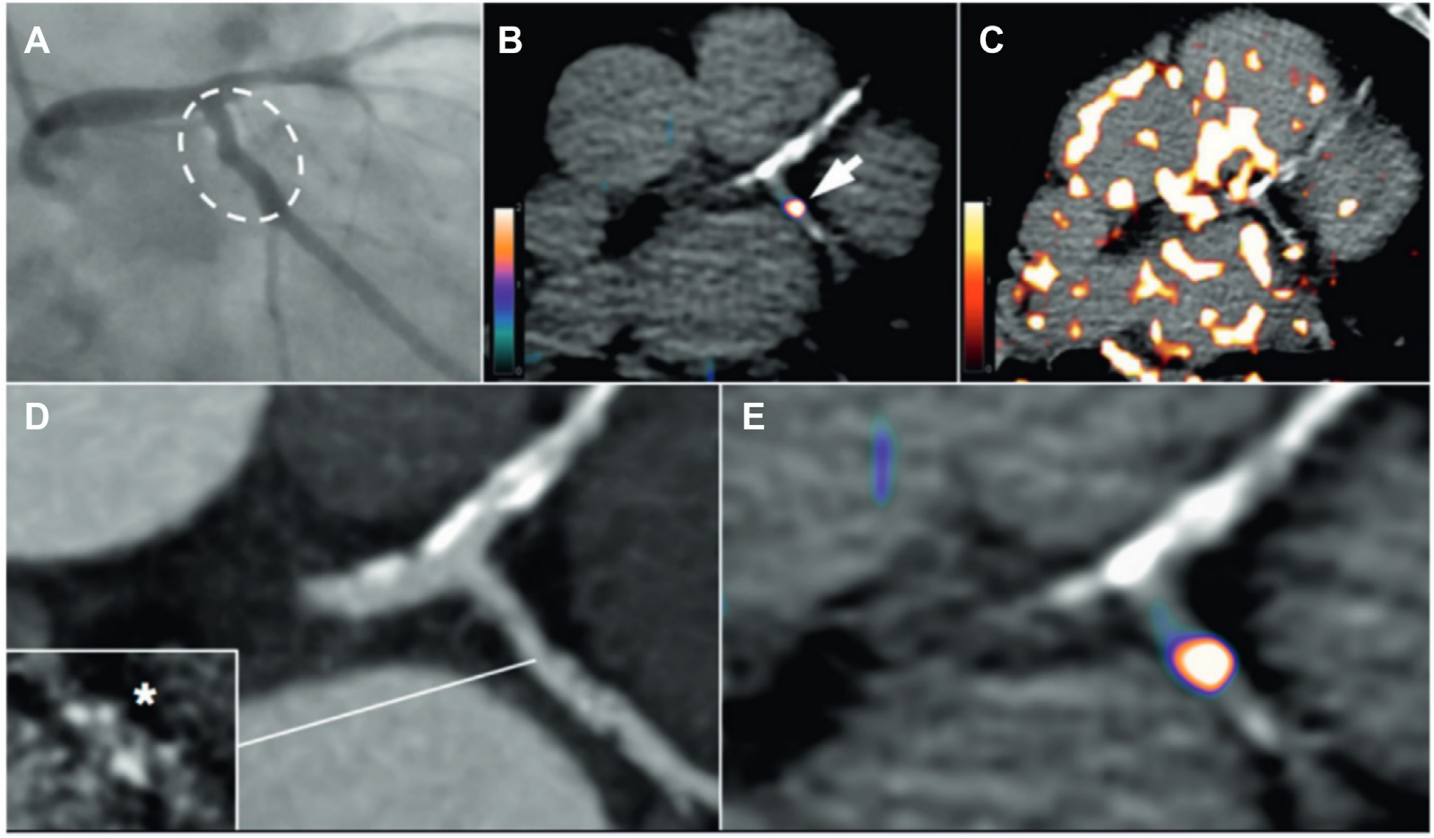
Coronary Artery PET Inflammation Imaging With 68Ga-DOTATATE Radiograph (A) and CT coronary angiograms (D) of a 67-year-old man with stable angina, showing minor left circumflex atheroma (hatched oval) with spotty calcification ([inset] ∗calcium scan) and calcified plaque in the left anterior descending artery. Although gallium-68-labeled DOTATATE (^68^Ga-DOTATATE) positron emission tomography (PET) (B, E) allows unimpeded interpretation of inflammation in the left circumflex artery lesion (B, arrow), and lack of signal in the left anterior descending artery, coronary ^18^F-fluorodeoxyglucose imaging is obscured by patchy myocardial tracer uptake (C). Adapted with permission from Tarkin et al.^93^
